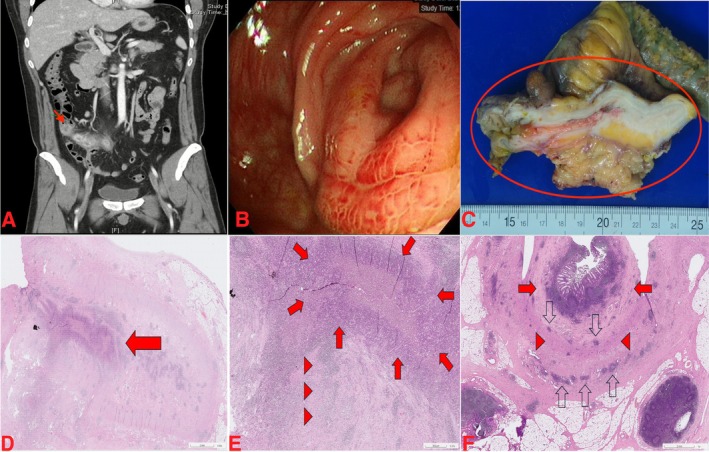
(A) Abdominal CT revealed an appendiceal mass with peri‐appendiceal fat stranding, cecal wall thickening (arrow), and regional lymphadenopathy. (B) Colonoscopy showed cecal inflammation without a discrete mass. (C) Intraoperatively, a 2 × 1 cm ulcerative mass (circle) confined to the appendix with partial ileal involvement. (D) Low power view of the appendix shows obliteration of the lumen and mucosa with marked inflammation (arrow). (E) Medium power view shows abscess formation in the appendiceal lumen with a neutrophilic cuffing (arrows) and mononuclear cell infiltrate in the muscular wall (arrow heads). (F) Another low power view shows submucosal fibrosis (arrows), muscular hypertrophy (arrow heads), and transmural lymphoid follicles both inner and outer the muscularis propria (hollow arrows).
